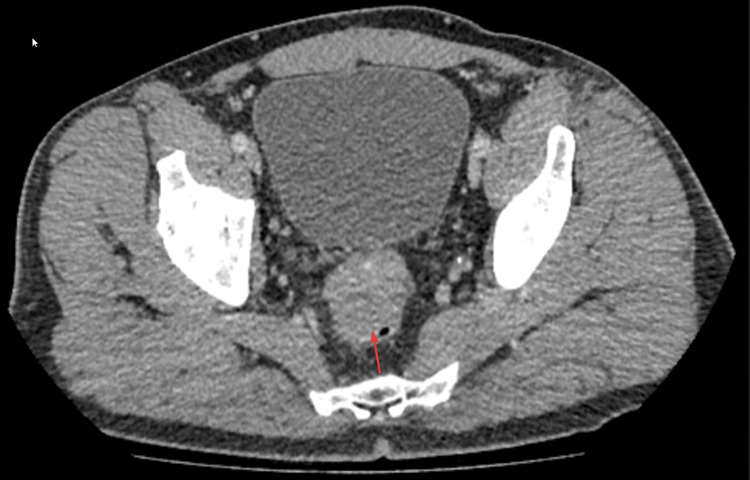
CT abdomen and pelvis with contrast done on May 4, 2024, showing a mass in relation to the upper aspect of the rectum at the rectosigmoid junction. The arrow indicates a 38.8 mm mass near the rectosigmoid junction distinct from the prostate and seminal vesicles and involving the bowel wall.
